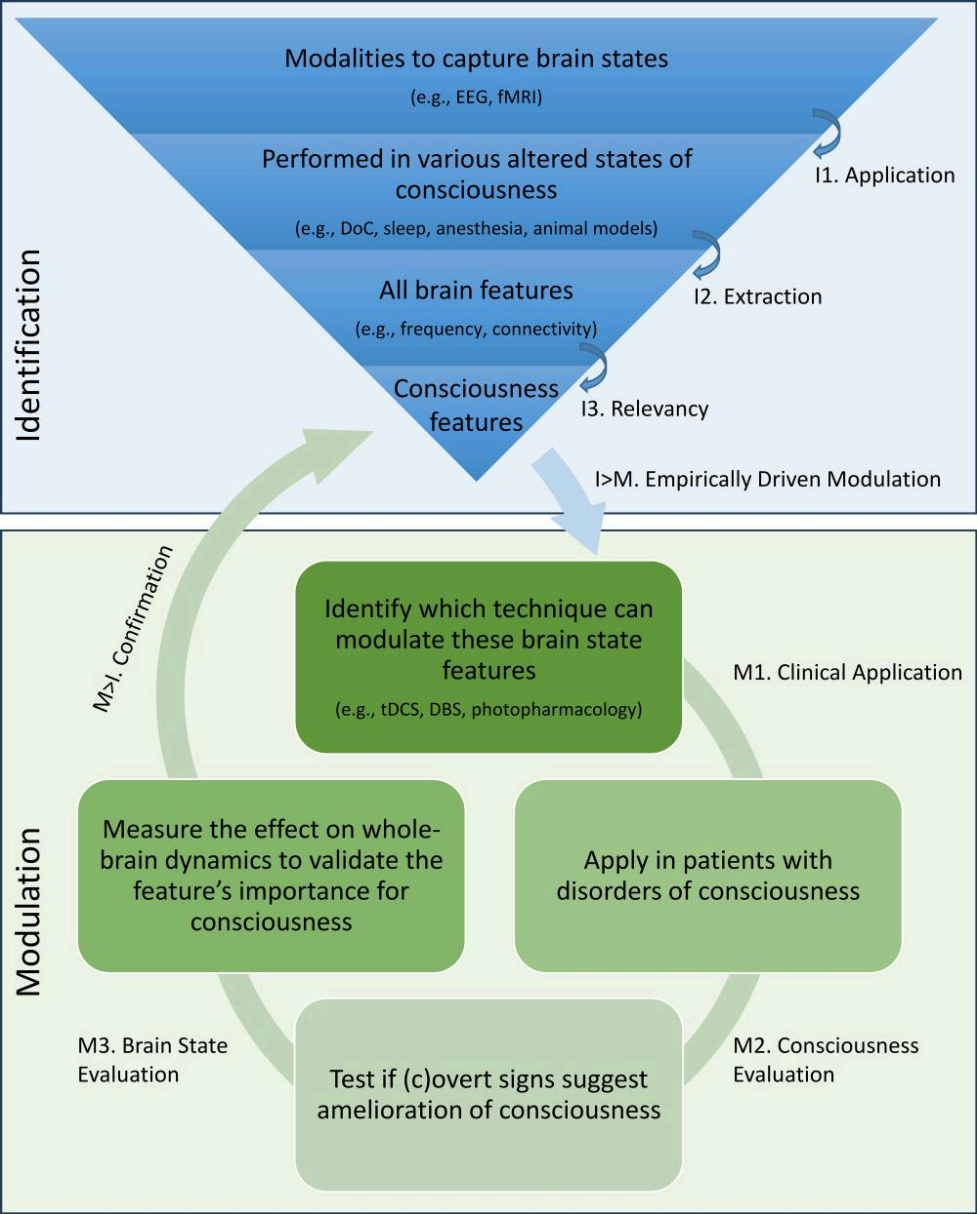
Therapeutic framework for identification and modulation of brain states. The framework consists of two parts: Identification and Modulation part that interact and support each other. Within each part, several levels of approaches can be distinguished, all of which are important for brain state research. From the top, the research focusing on brain state identification can utilize and develop techniques, such as electroencephalography (EEG) and magnetic resonance imaging (MRI), to capture the brain state, which can then be applied in various altered states of consciousness, such as disorders of consciousness (DoC) (*I1*), from which features can be extracted (*I2*), that can be evaluated on the relevancy for consciousness (*I3*). In the bottom part, modulation techniques, such as transcranial direct current stimulation (tDCS) and deep brain stimulation (DBS), can be identified to modulate these features, which could then be applied in patient populations (*M1*), where alterations in (c)overt signs of consciousness could be evaluated (*M2*), followed by an investigation on the whole-brain dynamics to examine brain state changes (*M3*). These two sides of the field are linked where successful manipulation of a feature, associated with amelioration of consciousness, could confirm its relevance as a feature of consciousness (*M > I*), and the other way around, relevant features could drive the direction of the neuromodulation field empirically (*I > M*). This framework is flexible enough to accommodate a variety of approaches and data types, but also provides enough structure to ensure consistency and rigour spanning from single unit recordings to whole-brain recordings in different levels of consciousness. It is adaptable to new discoveries and technologies as they emerge in various fields.
